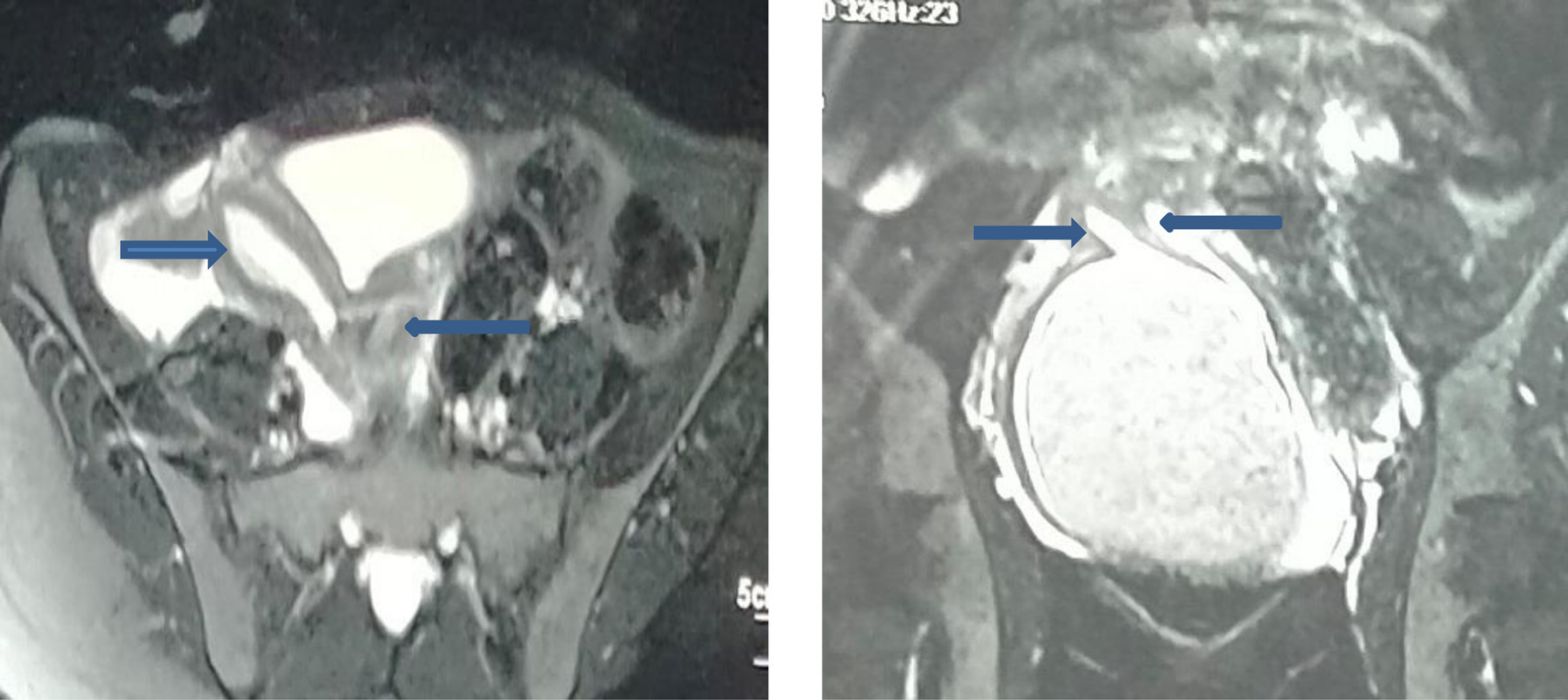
MRI Pelvis demonstrates, (A) uterus didelphys, (B) hematometrocolpos and 2 endometrial cavities.
